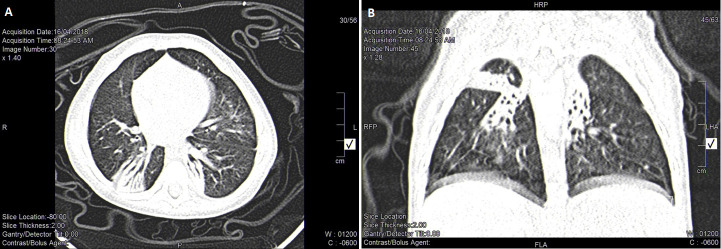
(A) Axial and (B) sagittal computed tomography images of the chest showing consolidation
